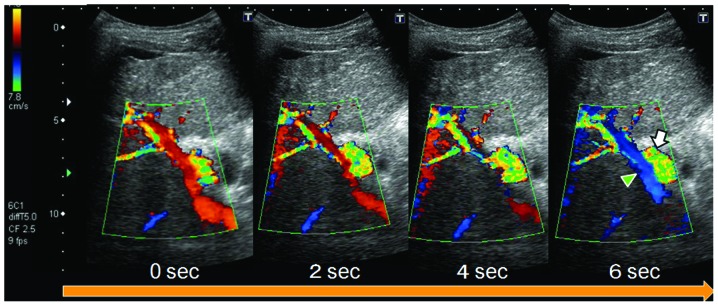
Changes in portal venous blood flow (arrow head) during deep inspiration. Color Doppler ultrasonography (US) images demonstrate the change in the blood flow from hepatopetal (red) to hepatofugal (blue) during deep inspiration breath-holding for 6 sec. The arrow indicates arterial blood flow. Images were acquired in February 2012.
